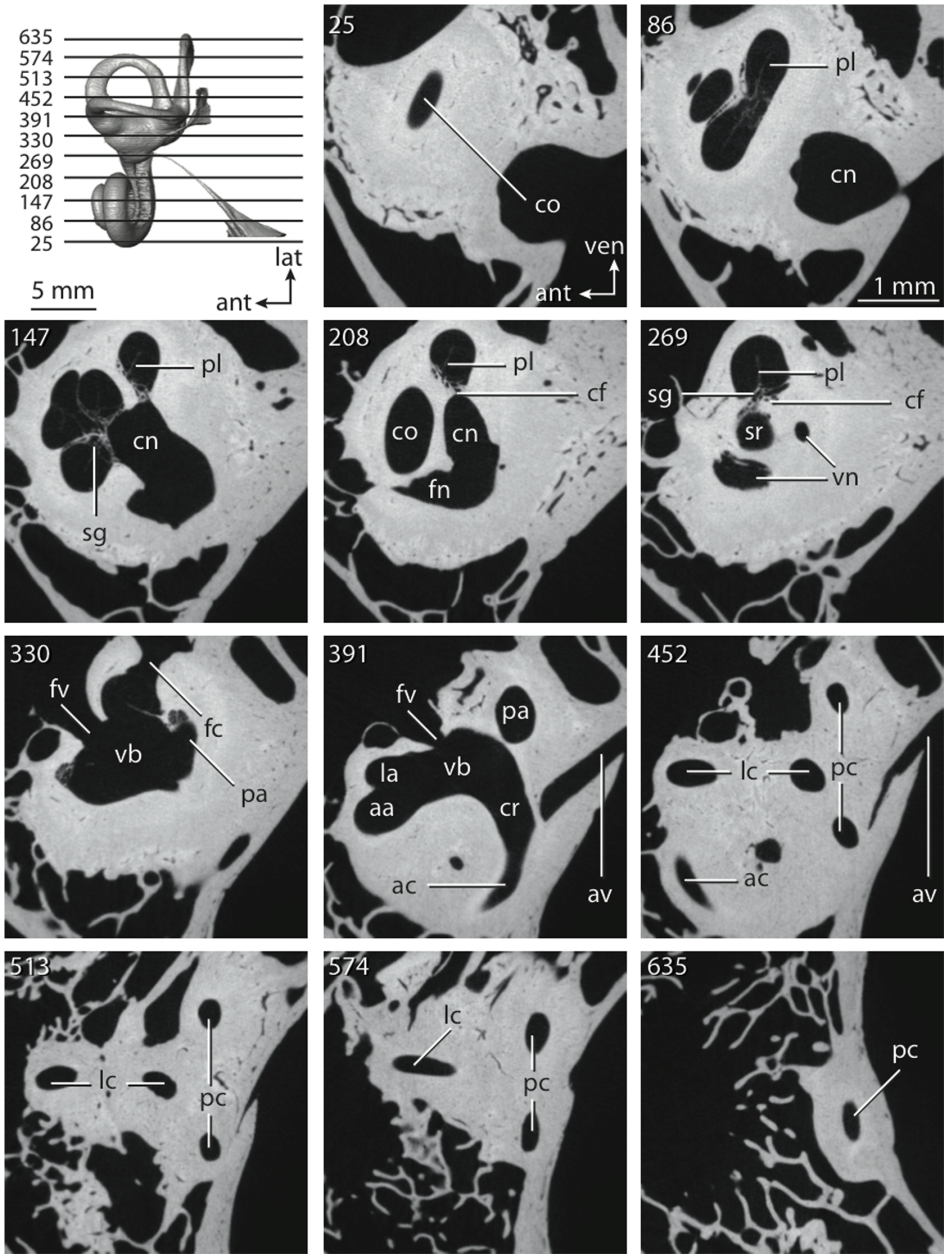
CT slices through ear region of*Homo sapiens*. Abbreviations listed at the end of the Materials and Methods section.
